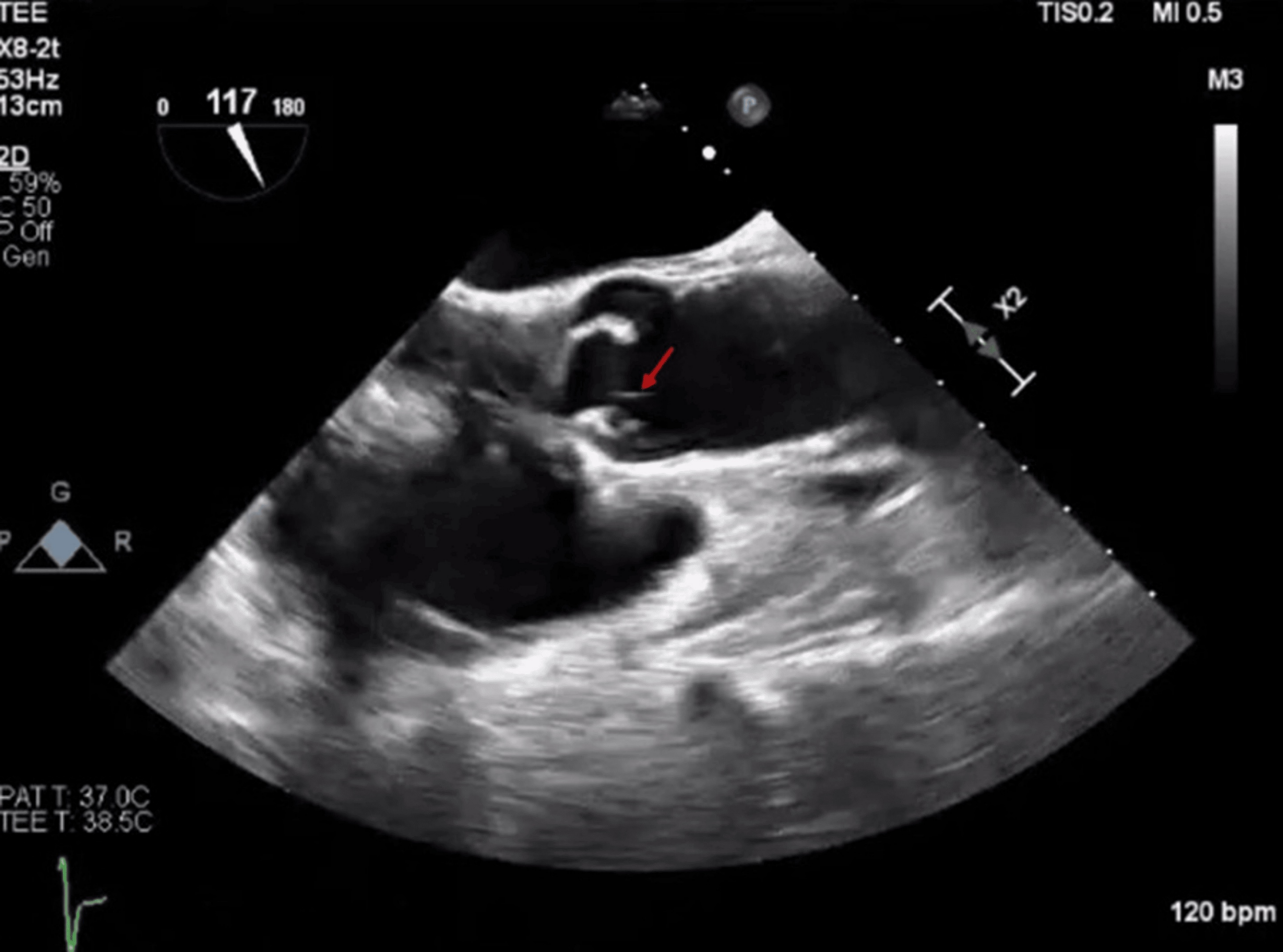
Transesophageal echocardiograph showed an ejection fraction (EF) of 50% to 55%, thrombus in the left arterial appendage, papillary fibroelastoma seen on aortic valve (red arrow), and mild mitral regurgitation
